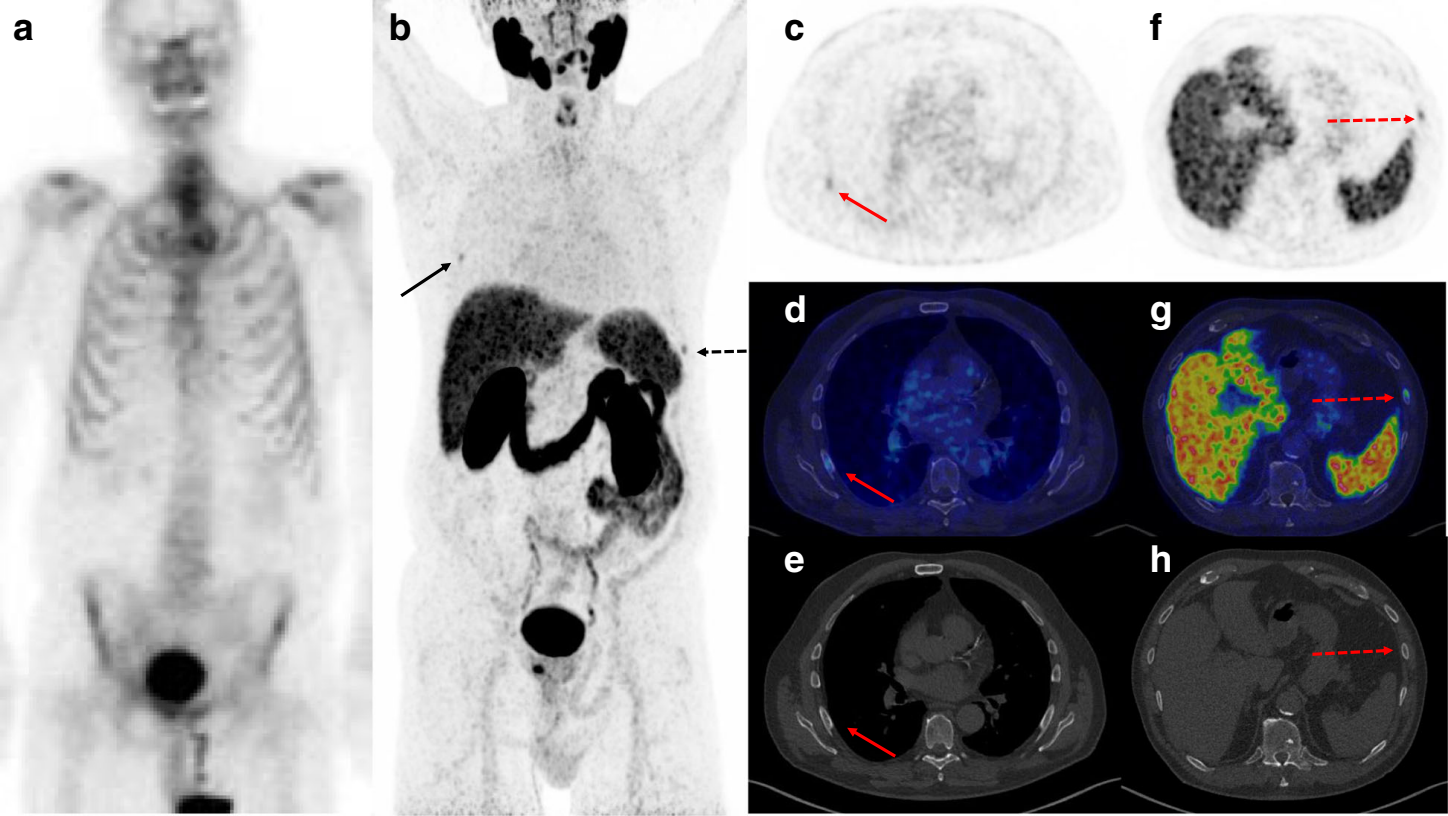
Example of a patient (PSA 13, Gleason score 9, T2b) with no bone metastases according to BS (shown in anterior projection) (a) but with two suspicious lesions in the seventh right rib and the eighth left rib according to the ^68^Ga-PSMA-11 PET/CT. Except for the ^68^Ga-PSMA-11 uptake in the ribs and the prostate, no other suspicious lesions were observed. The ^68^Ga-PSMA-11 PET MIP showed two rib lesions (b); the full arrow indicates a bone lesion in the seventh rib on the right side with corresponding axial ^68^Ga-PSMA-11 PET (c), ^68^Ga-PSMA-11 PET/CT fusion (d) and CT (e). The hatched arrow indicates a bone lesion in the left eighth rib with corresponding axial PSMA PET and corresponding axial ^68^Ga-PSMA-11 PET (f), ^68^Ga-PSMA-11 PET/CT fusion (g), and CT (h). The patient had no bone metastases according to the BVC: the patient had a radical prostatectomy without any systemic treatment, and the post-prostatectomy PSA level was < 0.1 ng/mL and remained so until this study was published
